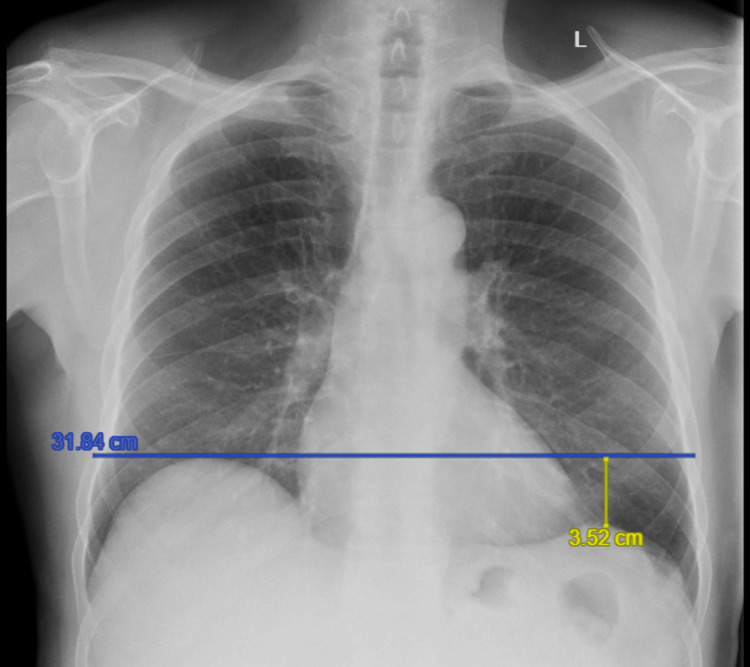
Chest X-ray in 2017, showing mild elevation of the right hemidiaphragm (blue line) when compared to the left hemidiaphragm (yellow line).
